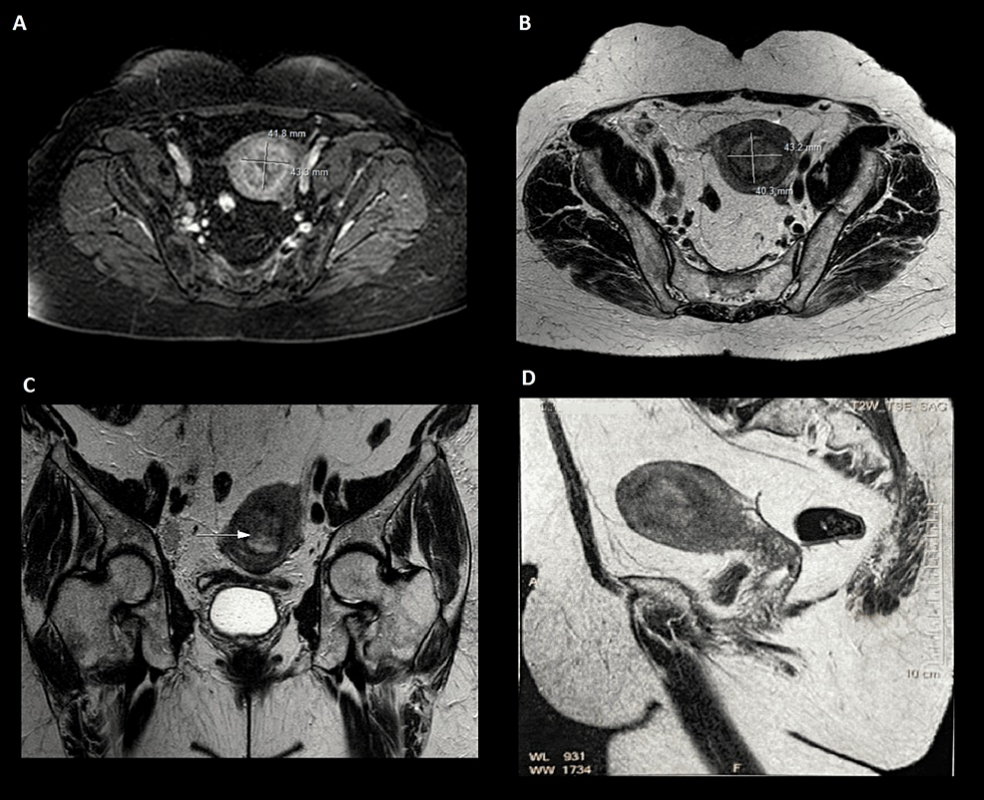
MR imaging of a 79-year-old woman with müllerian adenosarcoma of the uterus Axial T1 gado+ (A), axial T2 (B) coronal T2 (C), and sagittal T2(D) revealed endometrial tumor process (arrow), with heterogeneous hyper signal T2; after injection of contrast medium, there was moderate enhancement compared to myometrial enhancement.
